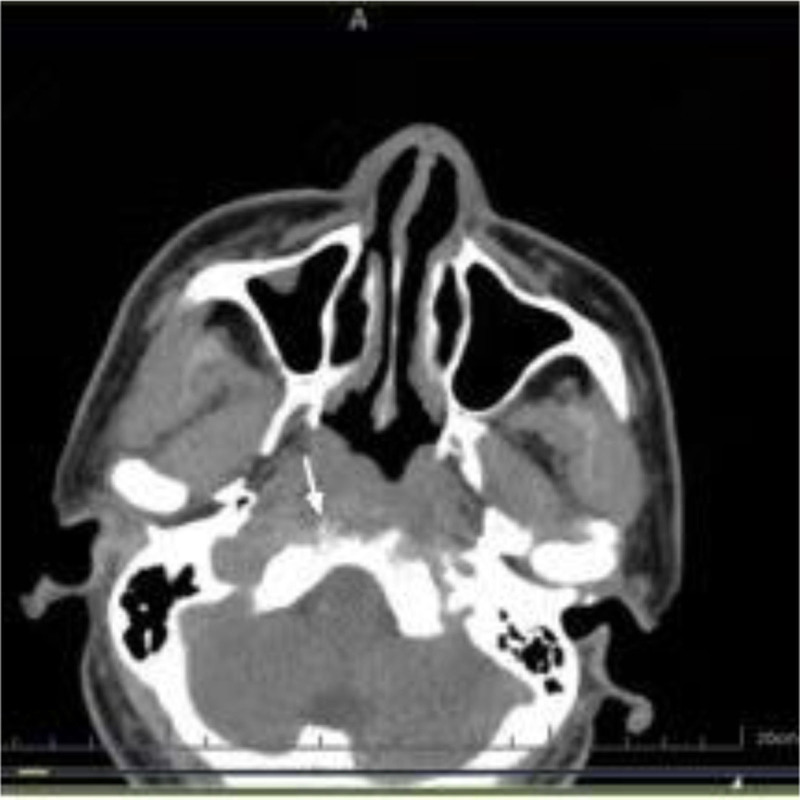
Nasopharyngeal CT: There is thickening of the soft tissue on the right side of the nasopharynx, with localized bone destruction in the right clivus. CT = computed tomography.
